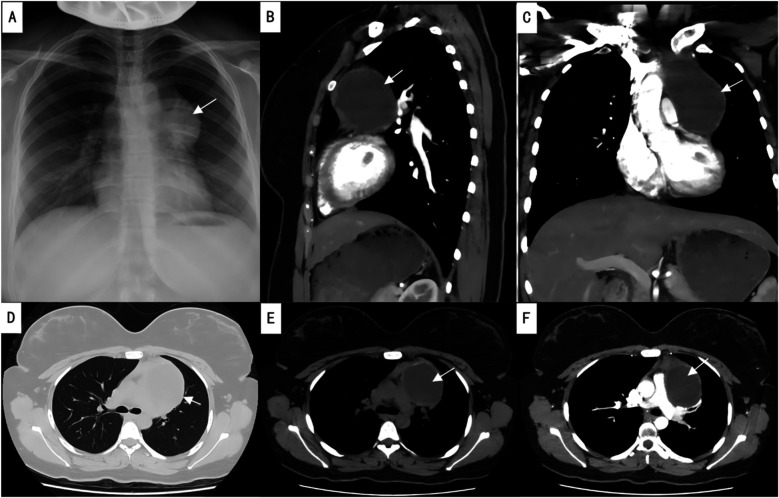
Preoperative imaging studies. (A) Preoperative chest x-ray; (B) sagittal view of contrast-enhanced chest CT; (C) coronal view of contrast-enhanced chest CT; (D) lung window of plain chest CT; (E) mediastinal window of plain chest CT; (F) axial view of contrast-enhanced chest CT. The arrows indicate the location of the lesion.
